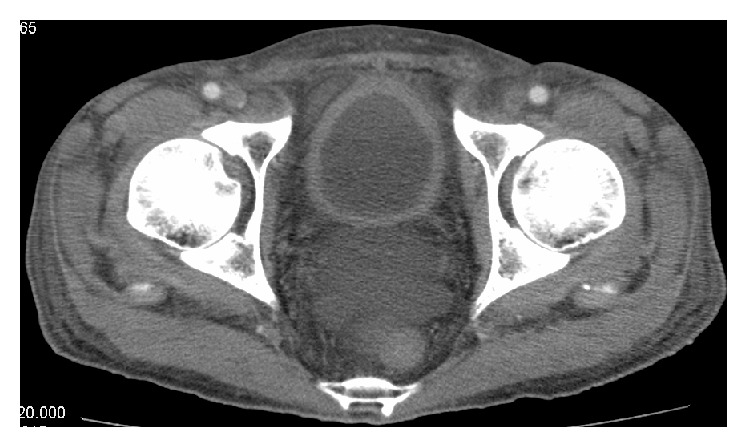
CT scan reveals bladder wall thickening.
